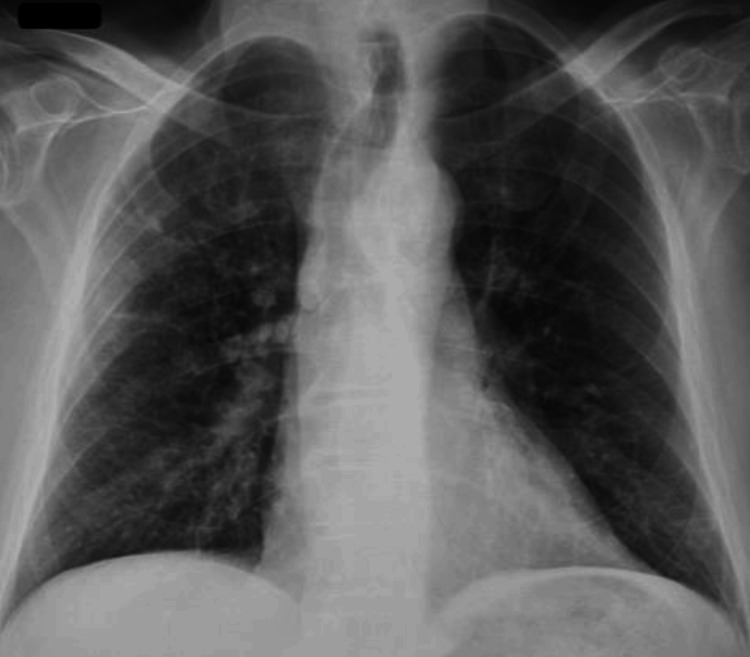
Chest X-Ray Official read: Diffuse interstitial opacities with suggestion of bibasilar ground-glass opacities. Finding may be seen with interstitial pneumonitis or mild pulmonary edema.
